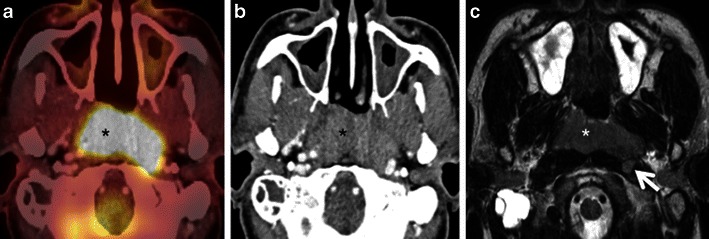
a Axial PET/CT image reveals a FDG-avid mass in the nasopharynx in keeping with a known nasopharyngeal carcinoma (*asterisk*). No separate retropharyngeal adenopathy is seen on this image. b Corresponding axial CECT image shows the infiltrative nasopharyngeal carcinoma (*asterisk*). No definite retropharyngeal adenopathy is seen. c Corresponding axial T2W MR image demonstrates the infiltrative nasopharyngeal carcinoma (*asterisk*) and an enlarged left retropharyngeal node (*white arrow*) suspicious for nodal metastasis. This node was missed on PET/CT due to its close proximity to the FDG-avid primary tumour and on CECT due to poor soft tissue contrast as compared to MRI. Detection of retropharyngeal lymph nodes in nasopharyngeal cancer affects TNM classification
